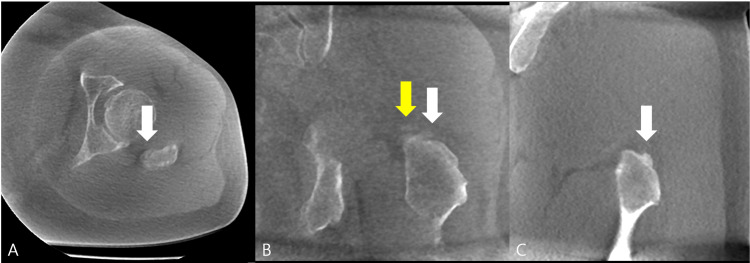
CT scan of the left hip. (A) Axial view demonstrating a bony spur (white arrow) arising from the posterior aspect of the greater trochanter.
